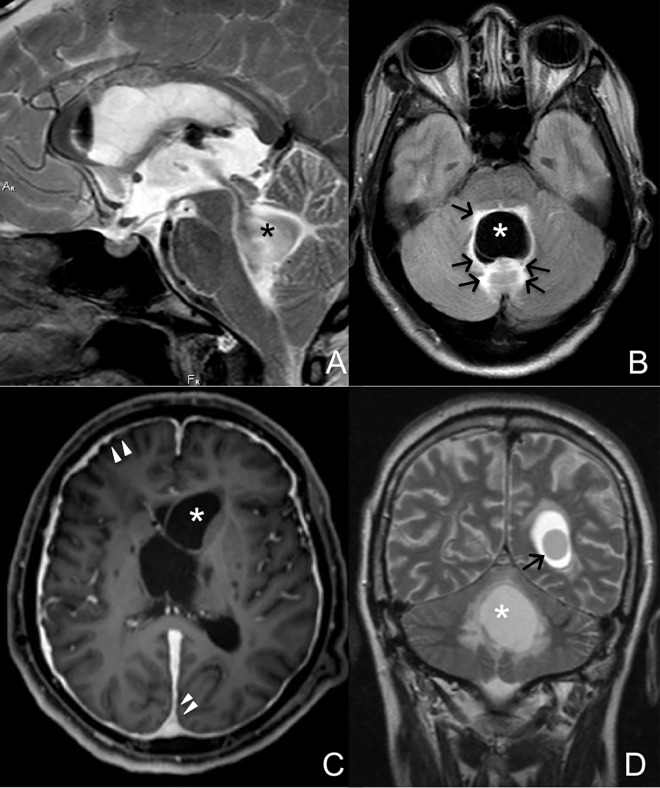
MRI images of cysts inside brain ventricles. The fourth ventricle is the most common site for ventricular neurocysticercosis. A large cyst (*) in the fourth ventricle (A) resulted in perilesional edema (arrows) in the patient’s posterior fossa (B). The lateral ventricles are also common sites of cyst location (C). Meningeal enhancement (arrowheads) is in a patient with a cyst (*) inside the left lateral ventricle. In some patients, multiple ventricles can be compromised. D, Cysts in the left lateral (arrow) and fourth (*) ventricles of a patient.
